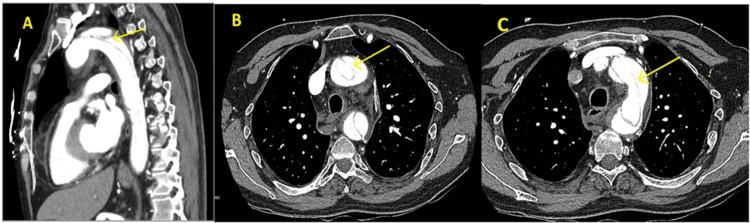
CT angiogram of the chest with contrast depicting Stanford type A dissection of the aorta (as indicated by the arrow).
